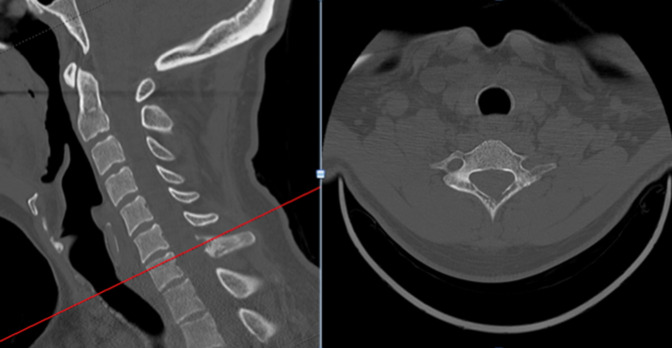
Sagittal and axial CT images of the cervical spine showing erosive changes to the C7 spinous process.
